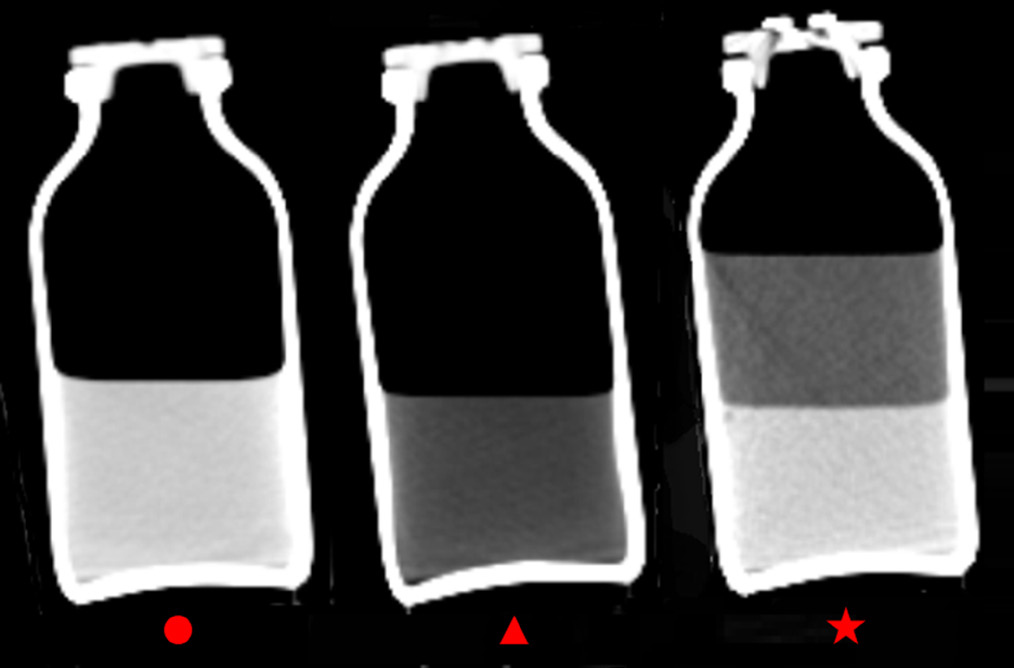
CT of water alone (circle), benzine alone (tringle), and a liquid mixed with benzine and water (star) [window width/window level = 520/–150]. Two separate fluid layers were observed in a mixture of benzine and water, with benzine floating on top of the water. The CT number of benzine was approximately −230 Hounsfield units.
